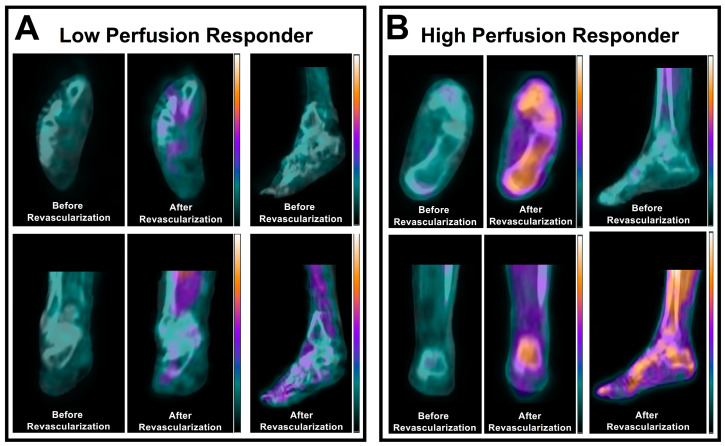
^99m^Tc-tetrofosmin SPECT/CT perfusion imaging before and after endovascular revascularization in (A) a low perfusion responder and (B) a high perfusion responder with CLTI. The patient with a high perfusion response to revascularization remained amputation-free for one year after intervention, while the low perfusion responder underwent an amputation procedure within one month of intervention [15].
